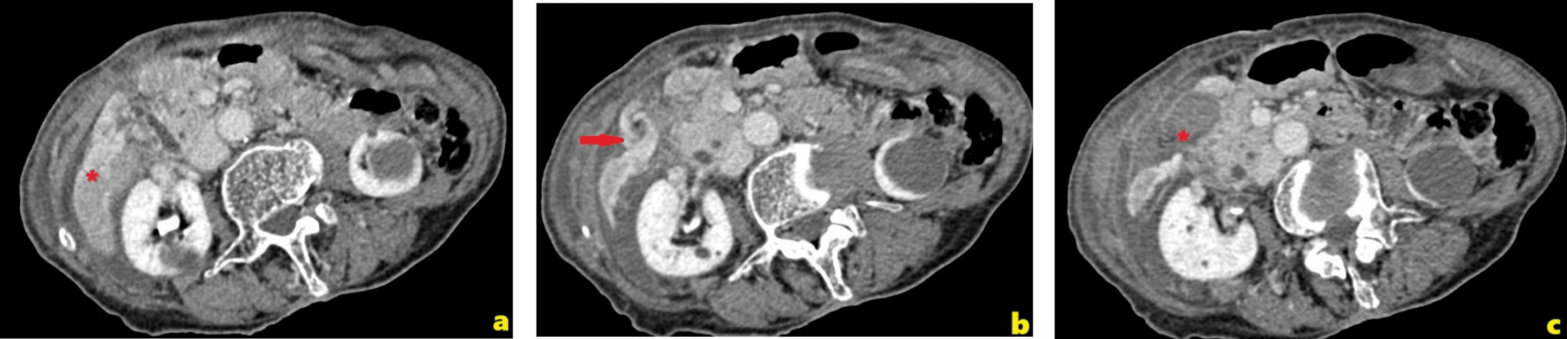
CT scan of the abdomen showing (a) part of the right liver parenchyma demonstrating perfusion disorder, (b) a whirlpool sign of the cystic pedicle, and (c) peritoneal fluid below the whirlpool sign CT: computed tomography
